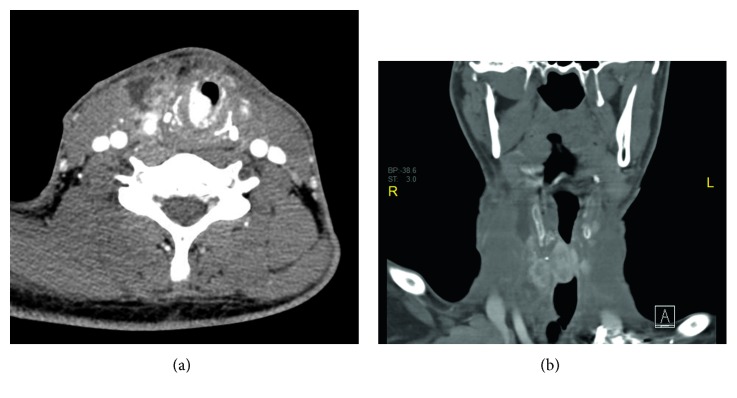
Contrast-enhanced CT showing subglottic soft tissue mass extending at the level of C4, C5, C6, C7 vertebrae, causing marked narrowing of the airways. (a) is axial view and (b) is coronal view.
